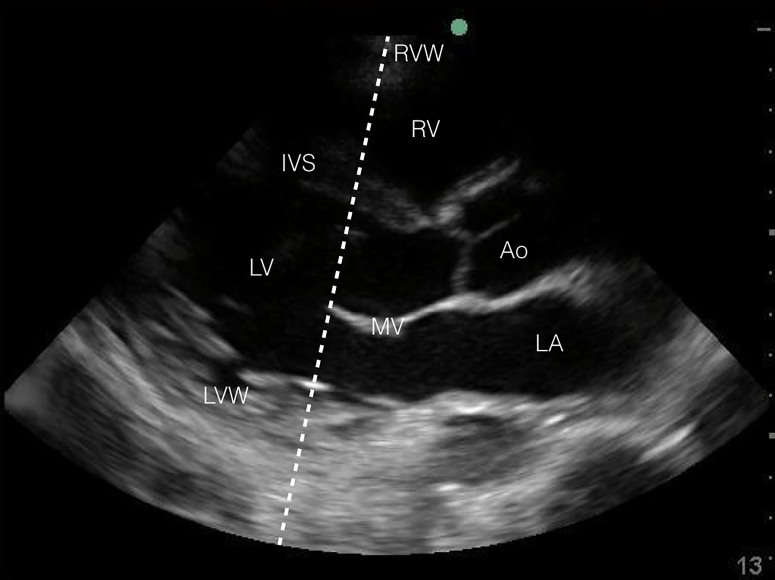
Normal comparison point-of-care transthoracic echocardiogram (parasternal long axis in 2D-mode) – Superimposed (dashed) line overlying the tip of the mitral valve indicating proper motion-mode striker placement. *RVW*, right ventricular wall; *RV*, right ventricle; *IVS*, intraventricular septum; *LV*, left ventricle; *Ao*, aorta; *MV*, mitral valve; *LVW*, left ventricular wall; *LA*, left atrium.
